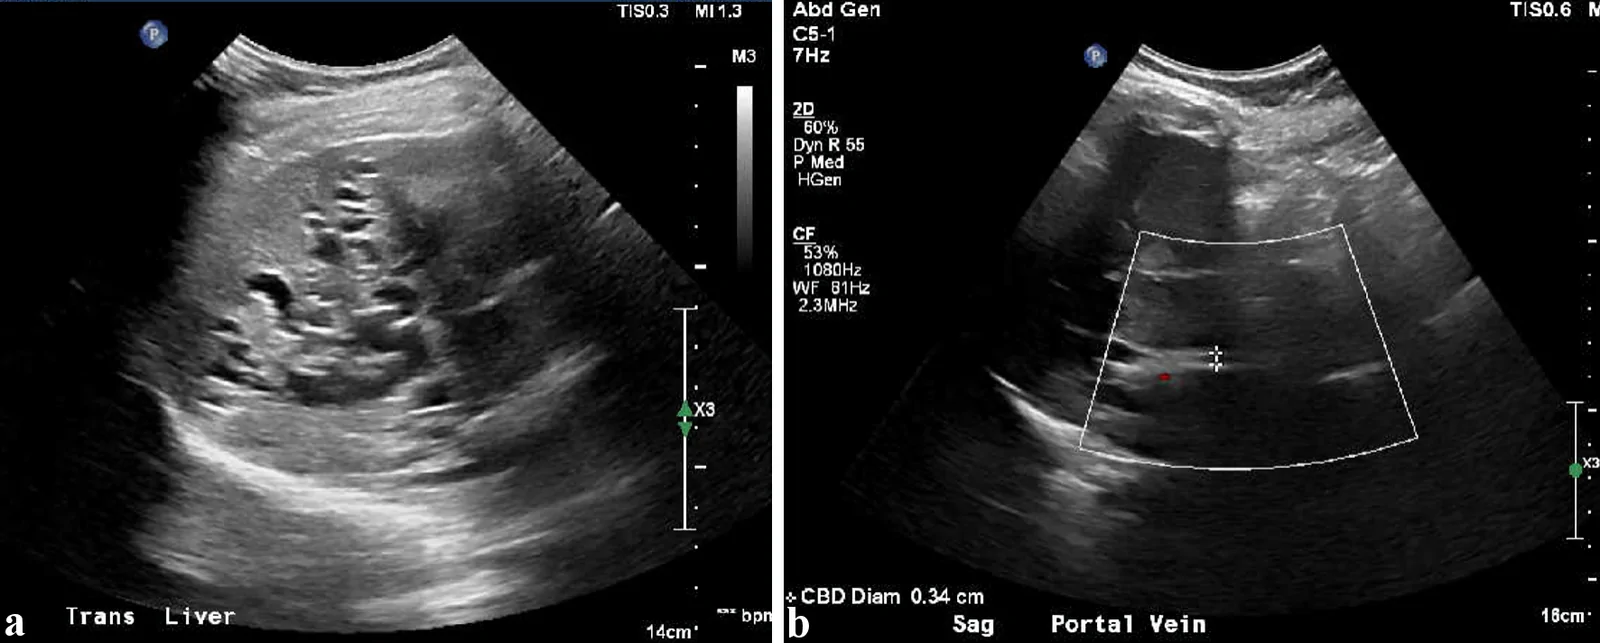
Ultrasound complete abdomen, showing (a) multiple cystic dilations in the liver and (b) common bile duct measuring 0.34 cm.
